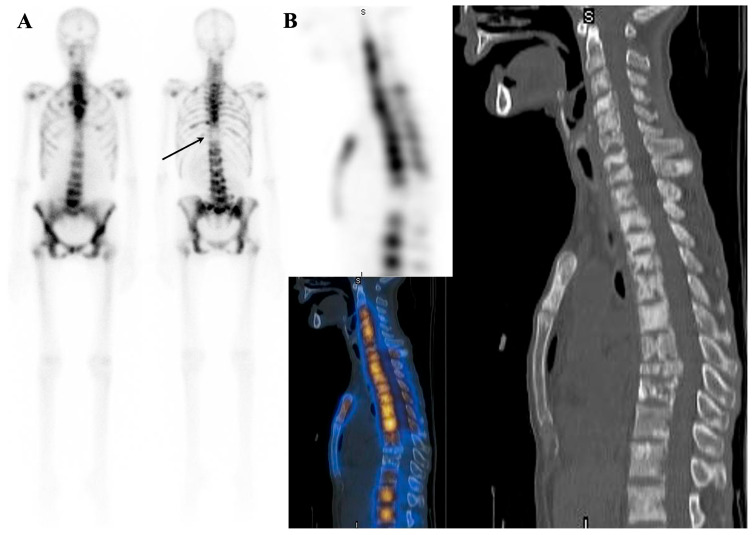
Atypical case of metastatic superscan in a 52-year-old woman with a history of breast cancer, prior radiation therapy, and recent paraplegia. In the recent bone scan, the patient exhibits multiple non-homogeneous foci of increased tracer uptake throughout the axial skeleton, accompanied by the absence of kidney visualization—a pattern consistent with a metastatic superscan (A). Additionally, a photopenic area is observed in the lower thoracic spine (arrow). Fused SPCET and CT images provide further insights, revealing a compression fracture in the T9 and T10 vertebrae, which coincides with the region previously treated with radiotherapy (B). This compression fracture exerts a compressive effect on the spinal cord.
